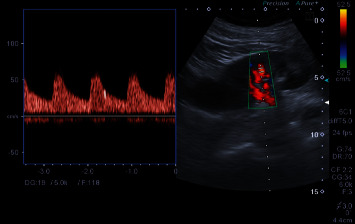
Spectral Doppler records HITS in a distal arterial branch of the allograft kidney. Embolic signal on renal Doppler recording of the distal arterial branch of the allograft kidney. The arrow indicates the high-intensity transient signals (HITS) seen suggesting the detection of a microemboli.
